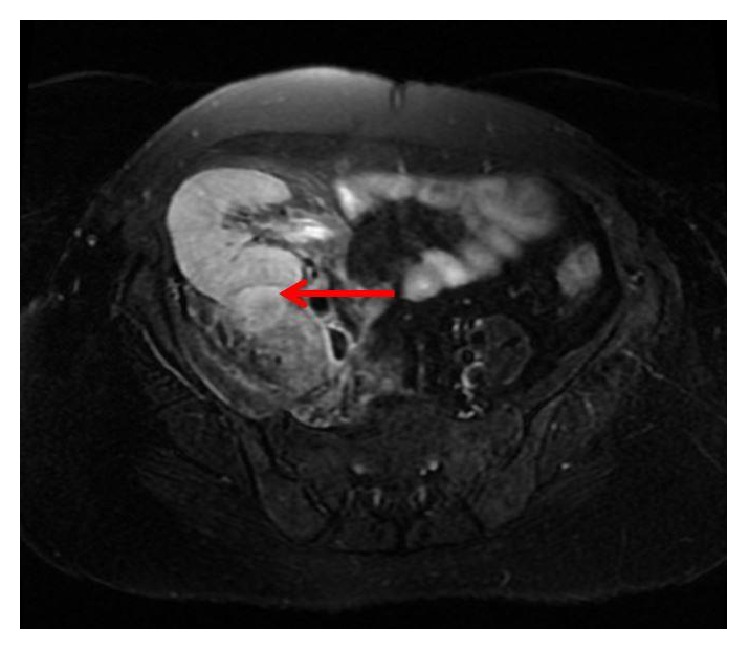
Contrasted T2-weighted magnetic resonance imaging. Right psoas abscess was in conjunction with the transplanted kidney. Ring-enhancement lesion was exhibited on magnetic resonance imaging (red arrow).
